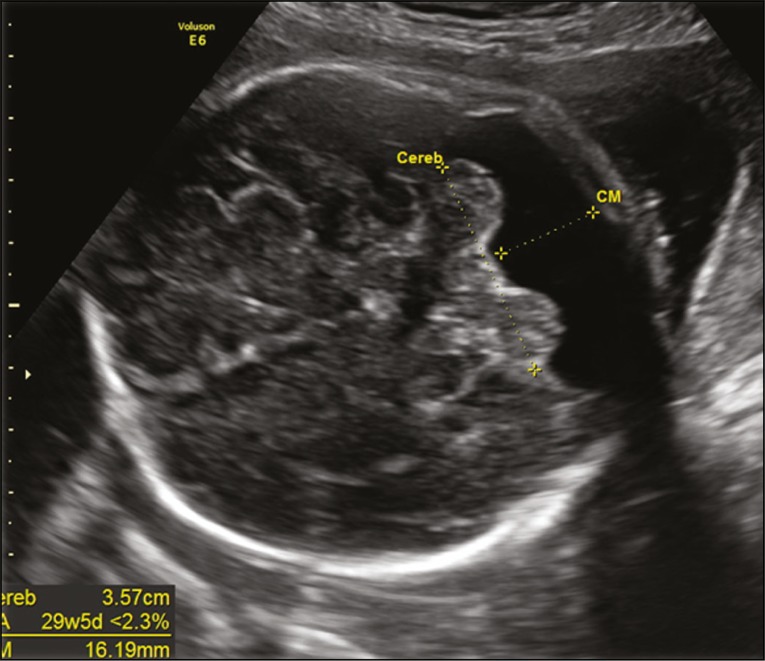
Ultrasound of a fetus with cerebellar hypoplasia, showing a transcerebellar diameter below the 10th percentile for gestational age. The cisterna magna may falsely appear to be increased because the cerebellum is small.
